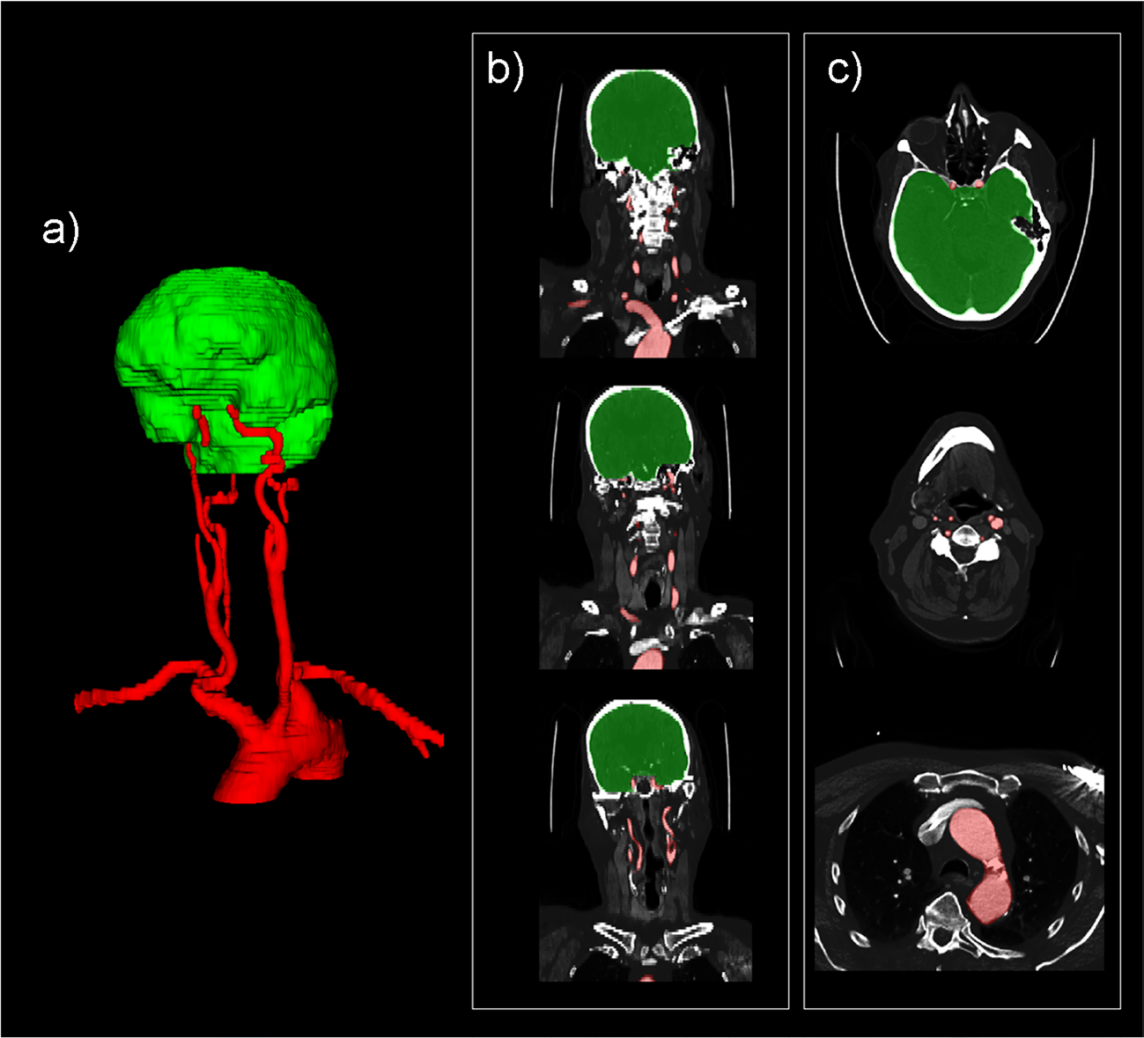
Example of a segmentation in the training set to selectively extract brain (including intracranial arteries) and extracranial arteries for focused view CTA. Step 1 concerned the segmentation of the cranial cavity and the proximal spinal canal until the level of the transition from the V4 to V3 segment of the vertebral arteries (green segmentation); step 2 concerned the segmentation of the aortic arch, subclavian arteries, common carotid arteries, proximal external carotid arteries, internal carotid arteries until the level of the transition from the cavernous to the cerebral segment, and vertebral arteries until the transition from the V3 to V4 segment (red segmentation). Segmentations in three-dimensional view (a), on some coronal slices (b), and on some axial slices (c) are shown
